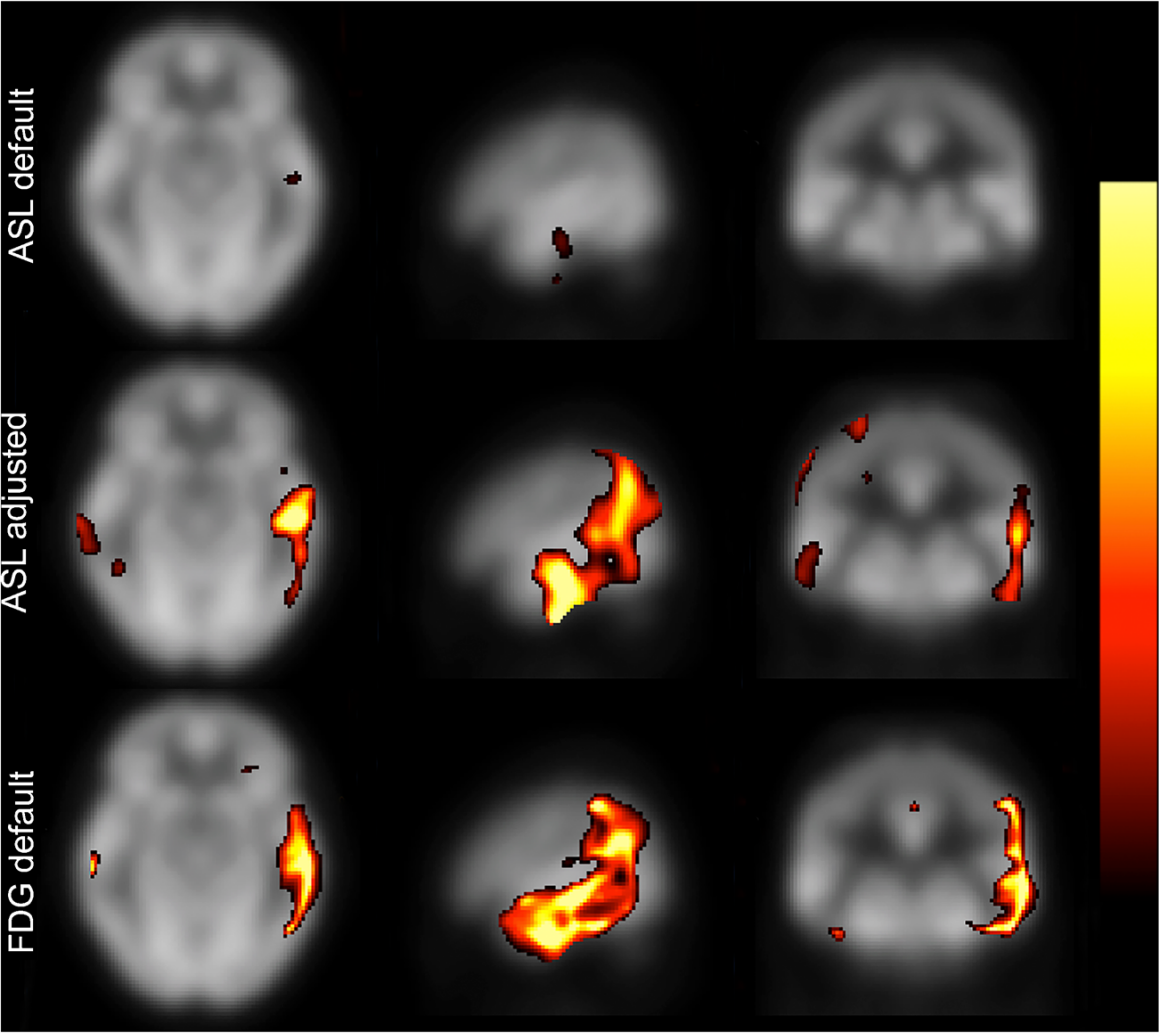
Representative discordant case. The ASL-based image of this AD case (Age: 67 years, MMSE: 25) was assessed as false negative by three of four readers, while the corresponding FDG-PET image was unanimously correctly diagnosed. The top row shows the ASL-based image with the default Z-score thresholds (lower 2.0, upper 6.0). The middle row shows the same image after threshold adjustment (0.77, 1.87). The bottom row shows the FDG-PET-based images of the same subject, with default threshold settings (2.0, 6.0). This is a representative case of a disease-typical deficit pattern evident on ASL-based images only after individually adjusting the threshold. Images are shown in a dichromatic colour scale ranging from red (lower threshold) to yellow (upper threshold). *ASL* arterial spin labeling, *AD* Alzheimer’s disease, *MMSE* Mini-Mental State Examination, *FTD* frontotemporal dementia, *PET* positron emission tomography
